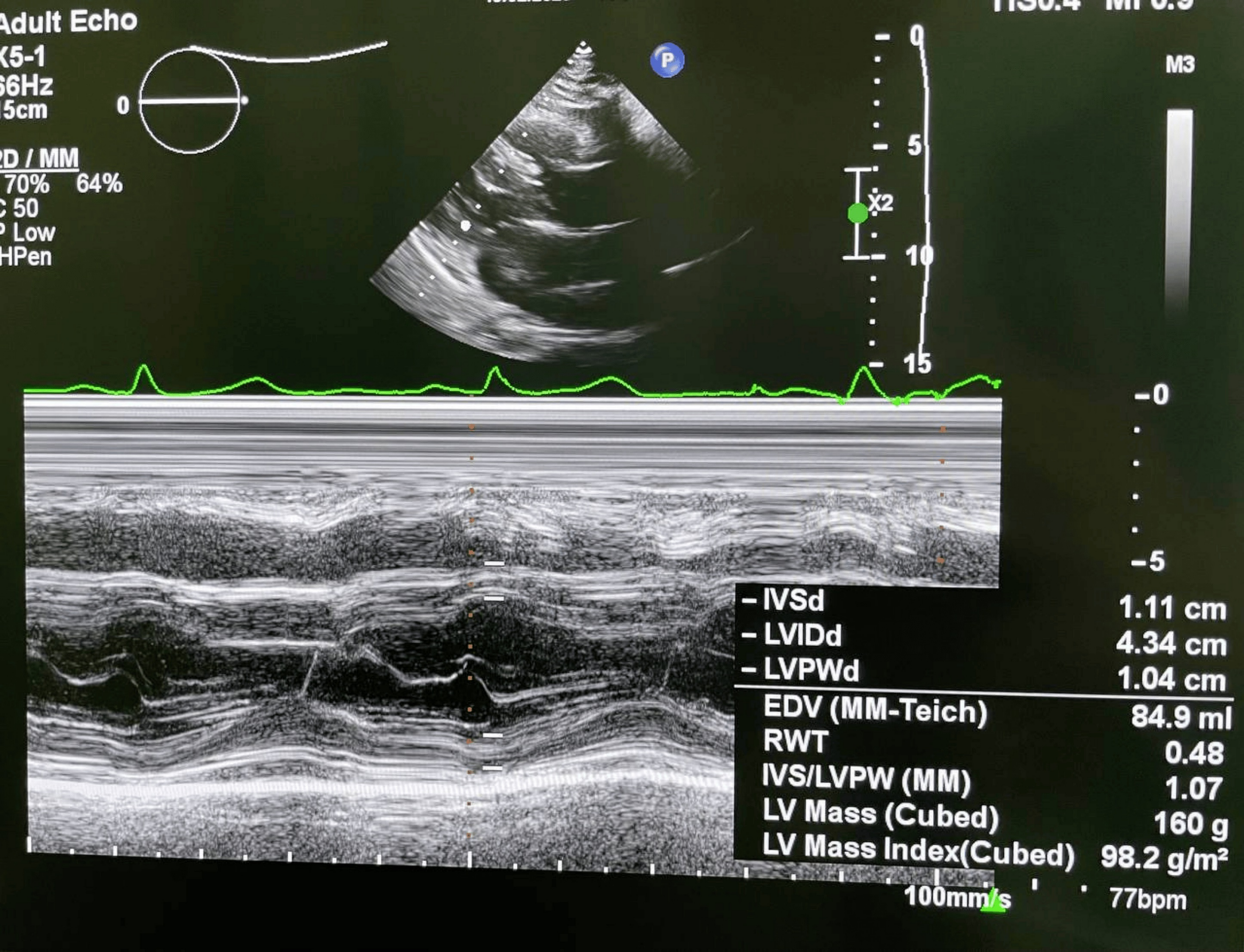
Parasternal long-axis M-mode echocardiogram. Measurements demonstrate normal interventricular septal thickness (IVSd), posterior wall thickness (LVPWd), and end-diastolic diameter (LVIDd), consistent with concentric remodelling. Left ventricular mass index is within the age-adjusted reference limits. No signs of congestive heart failure.
